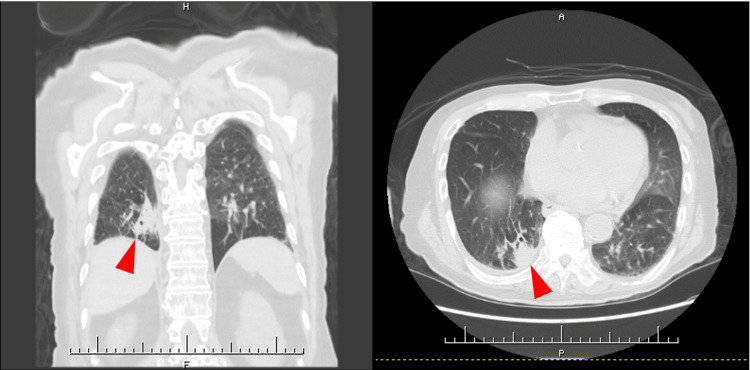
Chest computed tomography (CT) imaging on admission. Chest CT showing infiltration in the lower lobe of the right lung (arrowheads).
